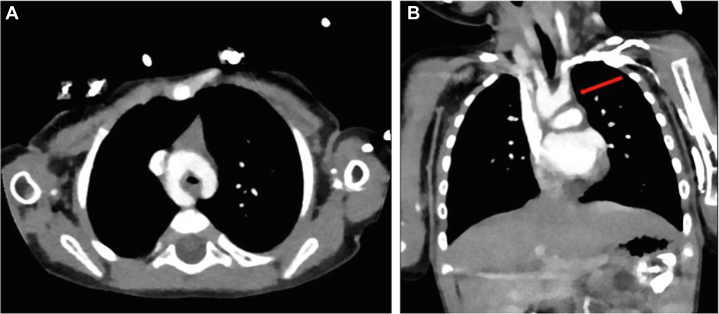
Computed Tomography Angiography Showing a Double Aortic Arch Forming a Complete Vascular Ring (A) Transverse view, and (B) coronal view highlighting the double arch (red arrow).
